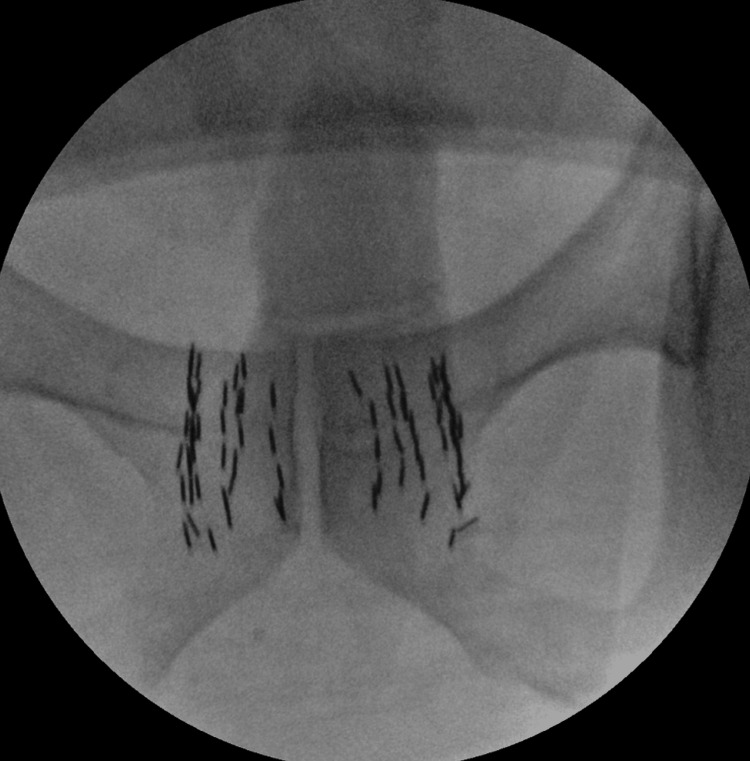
Confirmation of source distribution using postoperative X-ray fluoroscopy images.
